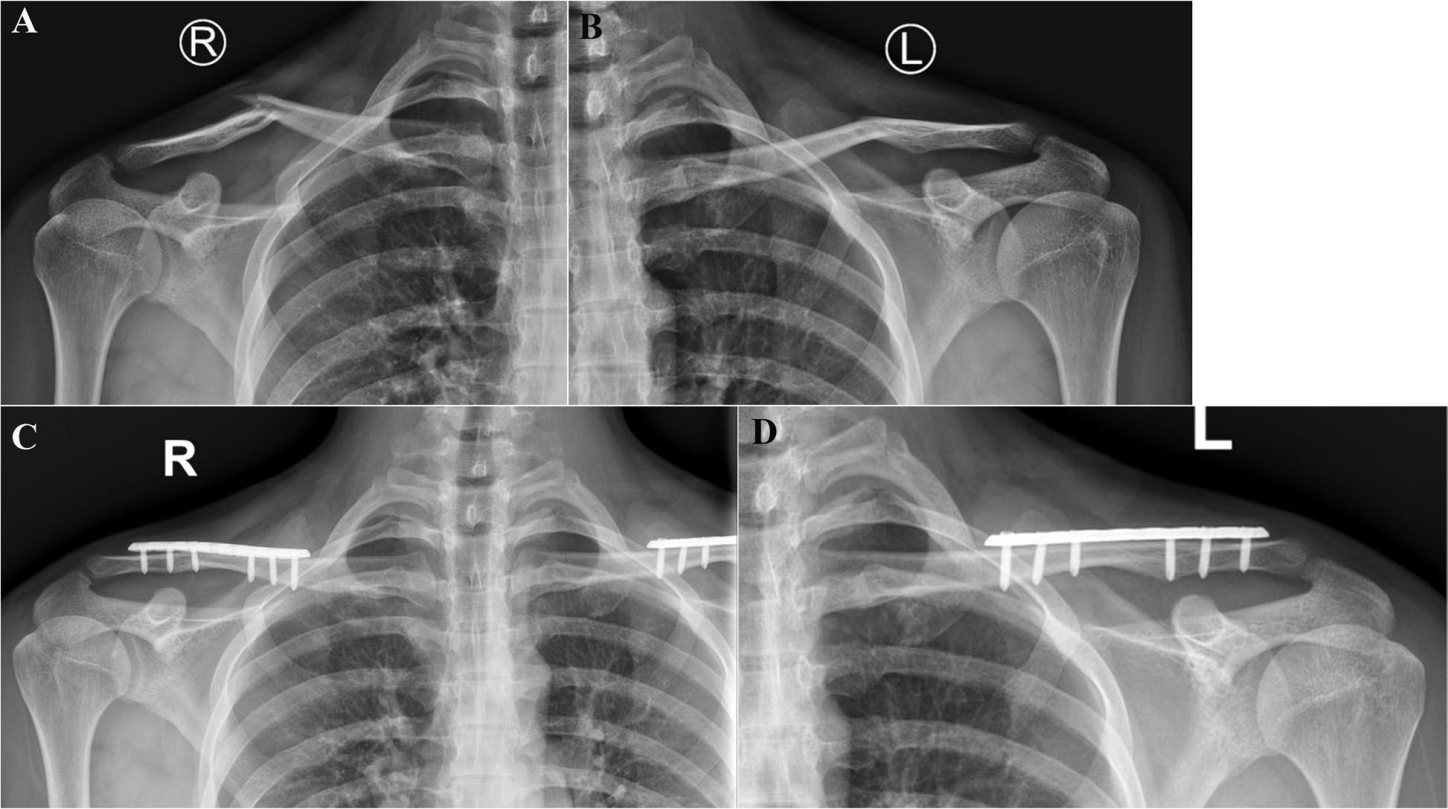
A, B Radiographs of an 18-year-old male patient showed bilateral displaced mid-clavicle fractures. C, D Follow-up radiographs at 6 months showed healing of the bilateral clavicle fractures after internal plate fixation on both sides
